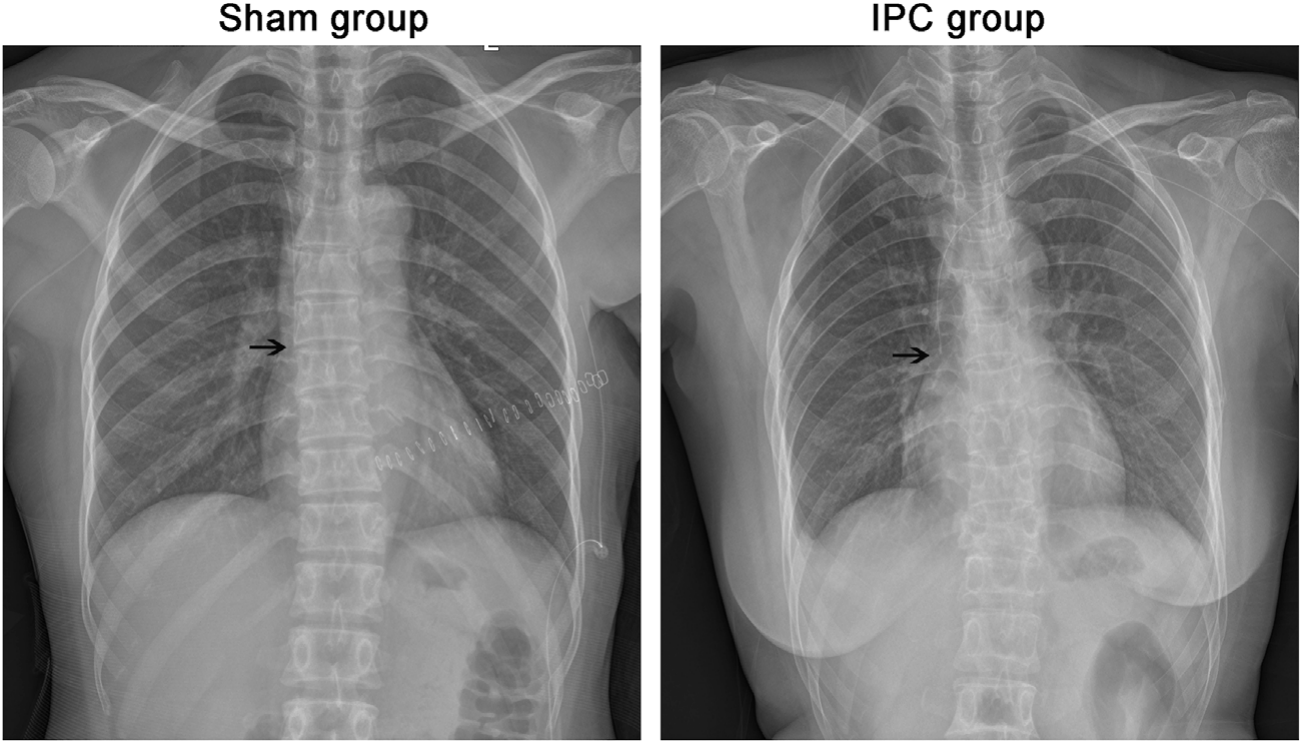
Evaluation of PICC. After the operation, all the cases were taken x-ray to determine the position of the catheter tip. The end of PICC catheter was in normal position between T5 and T7. The black arrow shows the end of the catheter.
